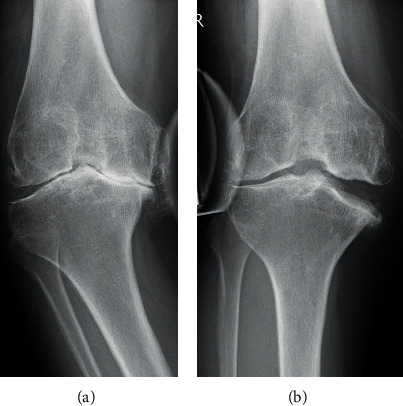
Stress radiography shows remarkable instability of the right knee. (Varus (a) and valgus (b)).
